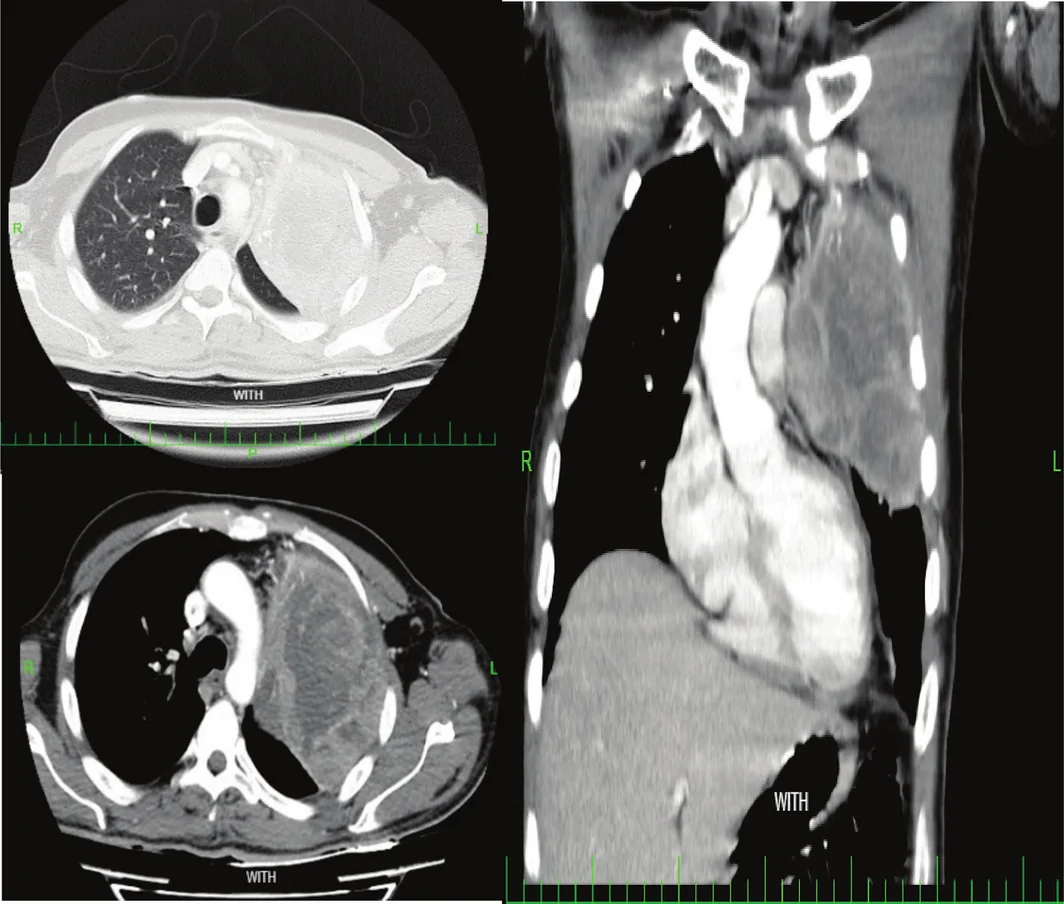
Contrast‐enhanced chest CT.
